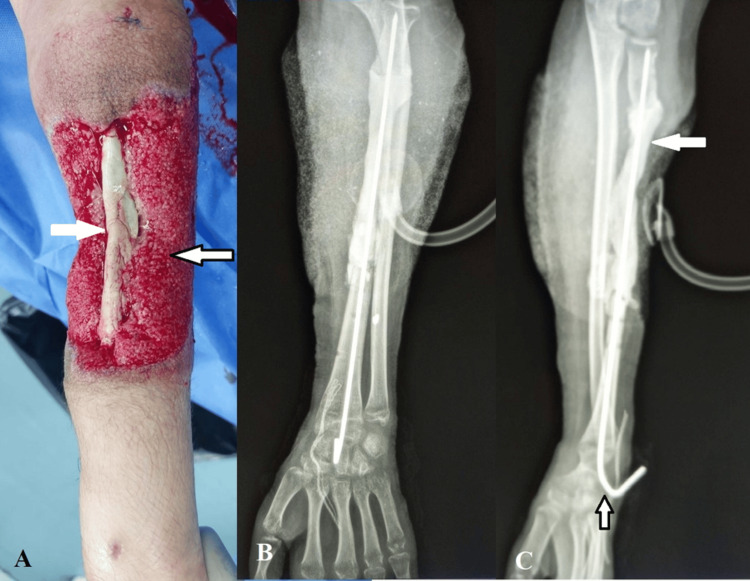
Granulation tissue formation following VAC therapy and bone cement application (A) Intraoperative photograph demonstrating healthy granulation tissue within the soft-tissue defect following application of VAC therapy and bone cement, used to fill the osseous gap between the proximal and distal bone ends and around the intramedullary fixation to promote induced membrane formation and aid infection control. (B, C) Postoperative anteroposterior (B) and lateral (C) radiographs showing intramedullary fixation with bone cement occupying the bone defect. VAC, vacuum-assisted closure
